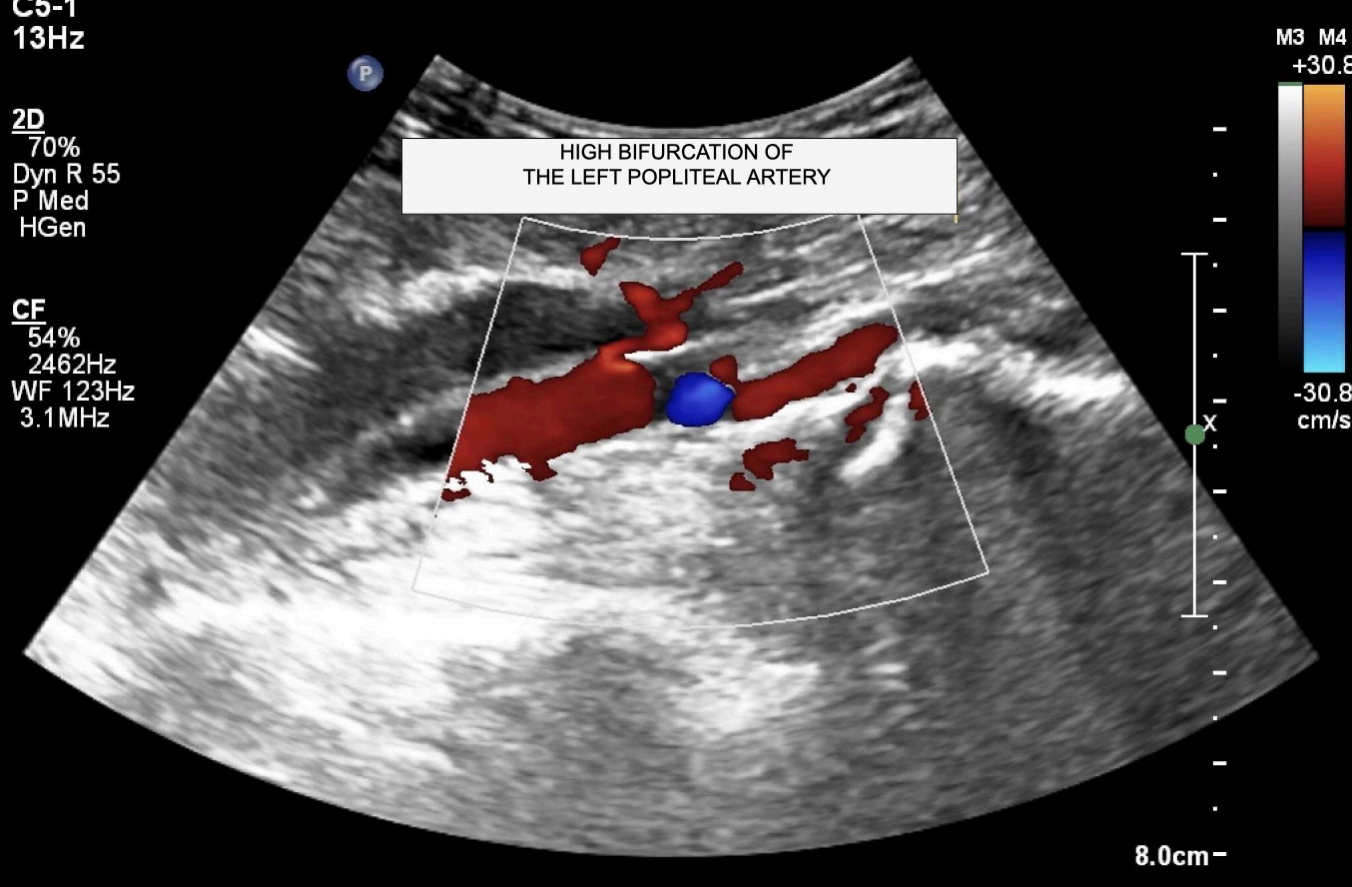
Doppler ultrasonography of the left lower limb in the longitudinal plane, showing high bifurcation of the popliteal artery.
